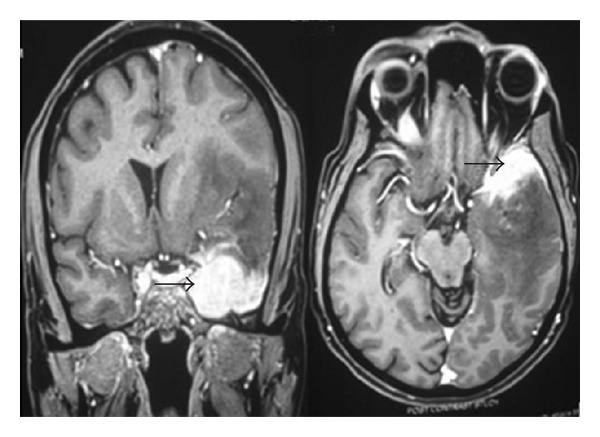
Coronal and Axial T1-weighted contrast image shows intensely enhancing extra-axial mass lesion extending from left cavernous area compressing and invading left anterior temporal lobe (black arrow). There is significant perilesional edema. Lateral ventricle is compressed with midline shift to the right.
